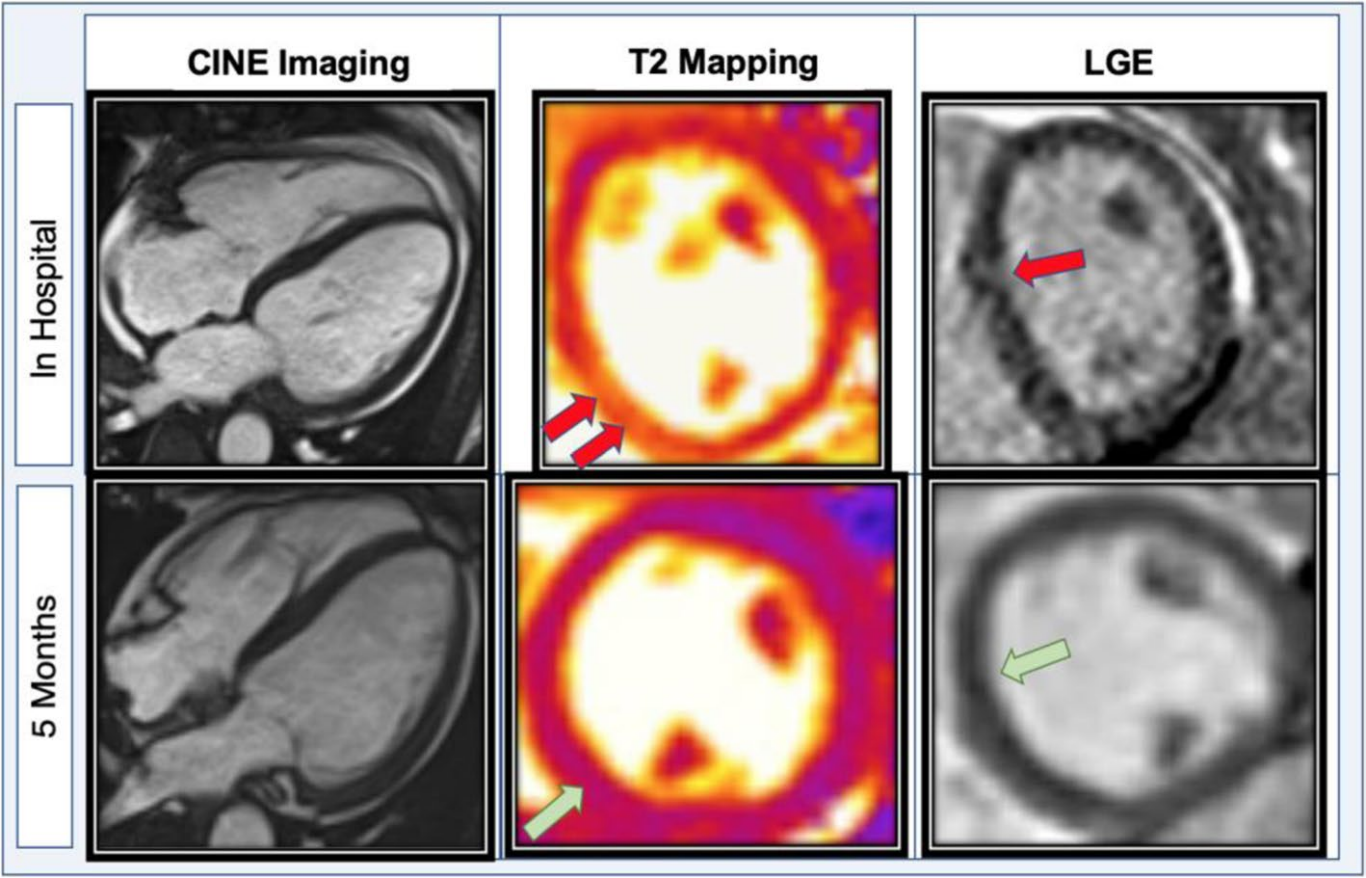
Cardiac magnetic resonance imaging (MRI) after six days of gilteritinib targeted therapy. After gilteritinib initiation, the patient developed significant clinical heart failure. Cardiac MRI revealed new left ventricular dilation and depressed systolic function with evidence of myocardial edema/ inflammation on T2 mapping (red arrows; max T2 of 70 milliseconds, normal < 53 ms), as well as patchy correlating non-ischemic patterned fibrosis with late gadolinium enhancement (LGE). Myocardial extracellular volume fraction was also elevated (not shown here). However, five months after therapeutic cessation, systolic function normalized with injury resolution (green arrows)
